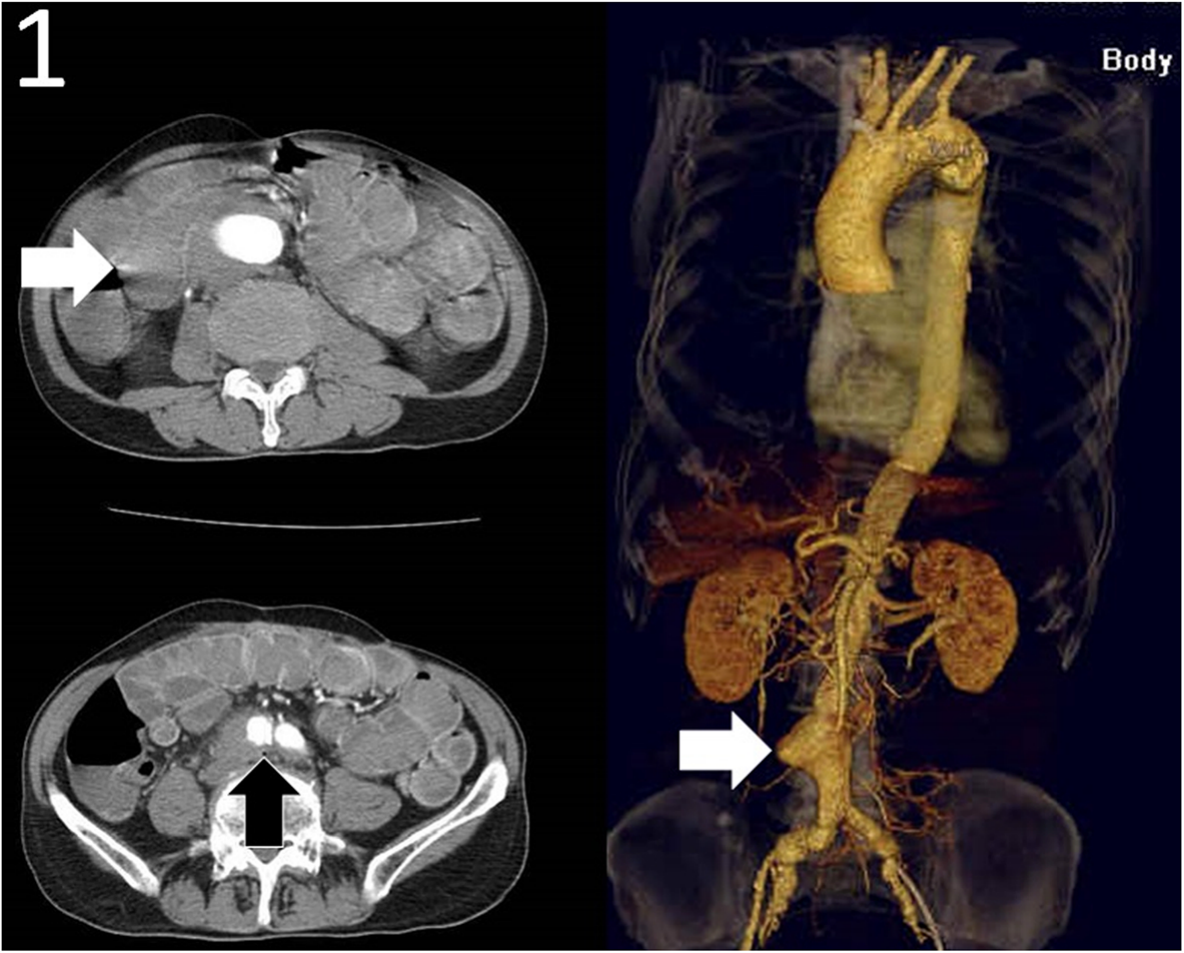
Computed tomographic angiography (CTA) of aorta depicted swollen and dilated bowel loop, contrast within bowel loop (white arrow), air bubble (black arrow), and an abdominal aortic aneurysm (AAA) (white arrow in reconstructed image of CTA)
